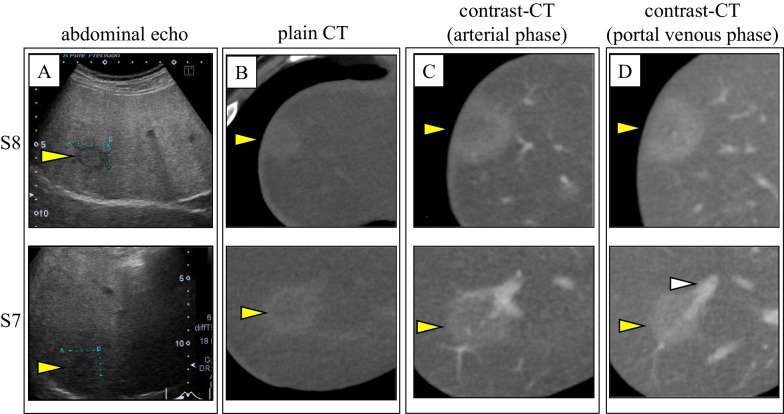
Ultra-sonographic (A), plain (B), and contrast computed tomography (CT) (C arterial phase; D portal venous phase) of the lesions. The upper images refer to the S8 lesion, and the images below refer to the S7 lesion. The yellow arrowheads indicate the lesions, and the white one indicates portal branch for S7
